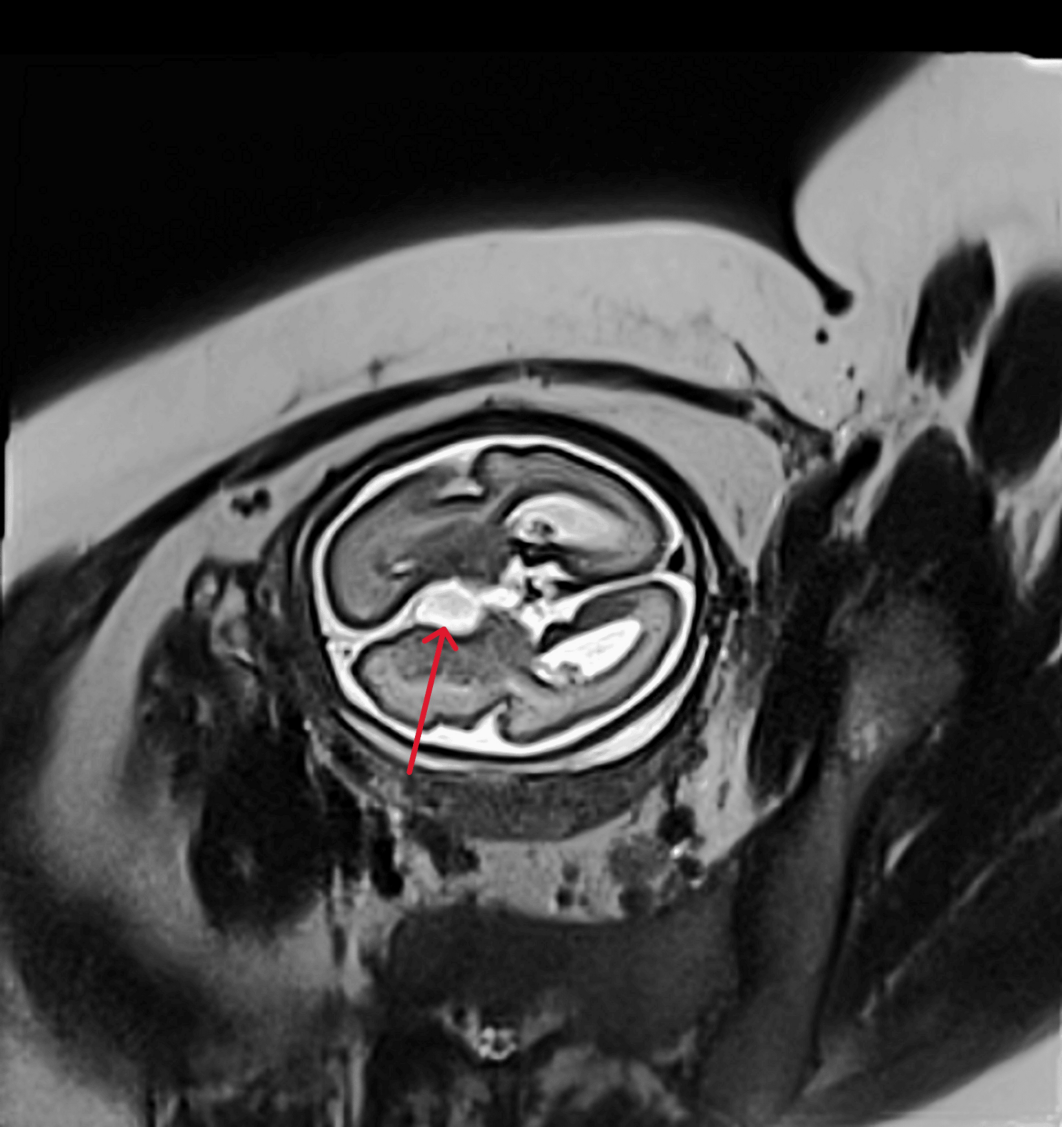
Fetal MRI: T2 sequence showing an interhemispheric arachnoid cyst (arrow)
